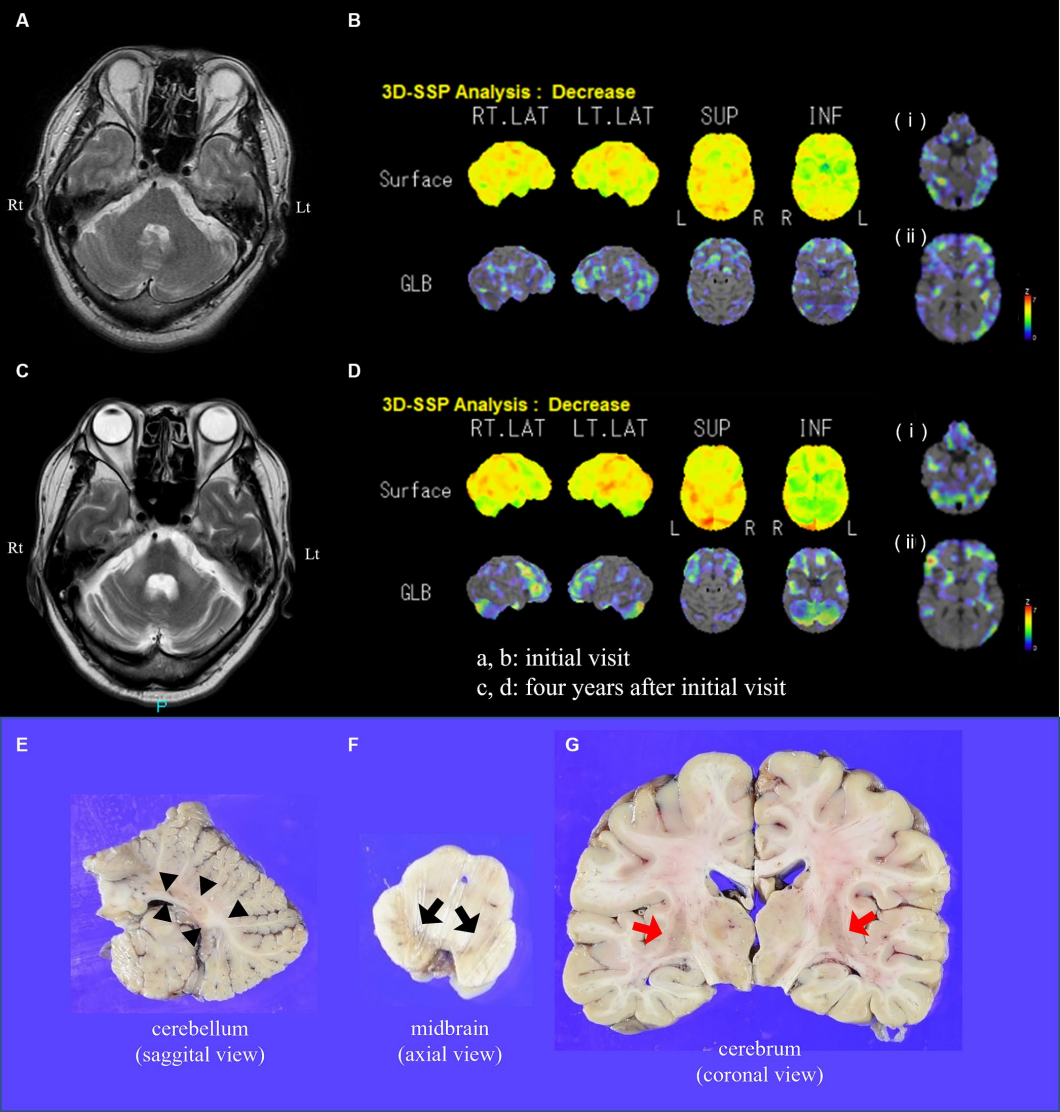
Brain MRI, perfusion imaging, and macroscopic appearance of the brain in the present case. At initial visit, (A) T2-weighted imaging on brain MRI and (B) three-dimensional stereotactic surface projection (3D-SSP) analyses on ^123^I-IMP SPECT at the level of (i) the brainstem and cerebellum and (ii) striatum reveal no evident abnormalities. Four years after initial visit, (C) T2-weighted imaging reveals marked cerebellar atrophy, hot cross bun sign, and high intensity of middle cerebellar peduncles. (D) 3D-SSP analyses on ^123^I-IMP SPECT reveal (i) decreased blood flow in the bilateral cerebellum and (ii) mild decreased blood flow in the right striatum. The sectional views of the cerebellum (E), midbrain (F), and cerebrum (G) are shown. (E) Modest atrophy, notably in the white matter (arrowheads), of the cerebellum is shown. (F) Severe depigmentation of the substantia nigra is evident (black arrows). (G) The putamen shows slightly brownish color and mild atrophy (red arrows). MRI, magnetic resonance imaging; ^123^I-iodoamphetamine single-photon emission computed tomography, ^123^I-IMP SPECT; L(t), left side; R(t), right side.
